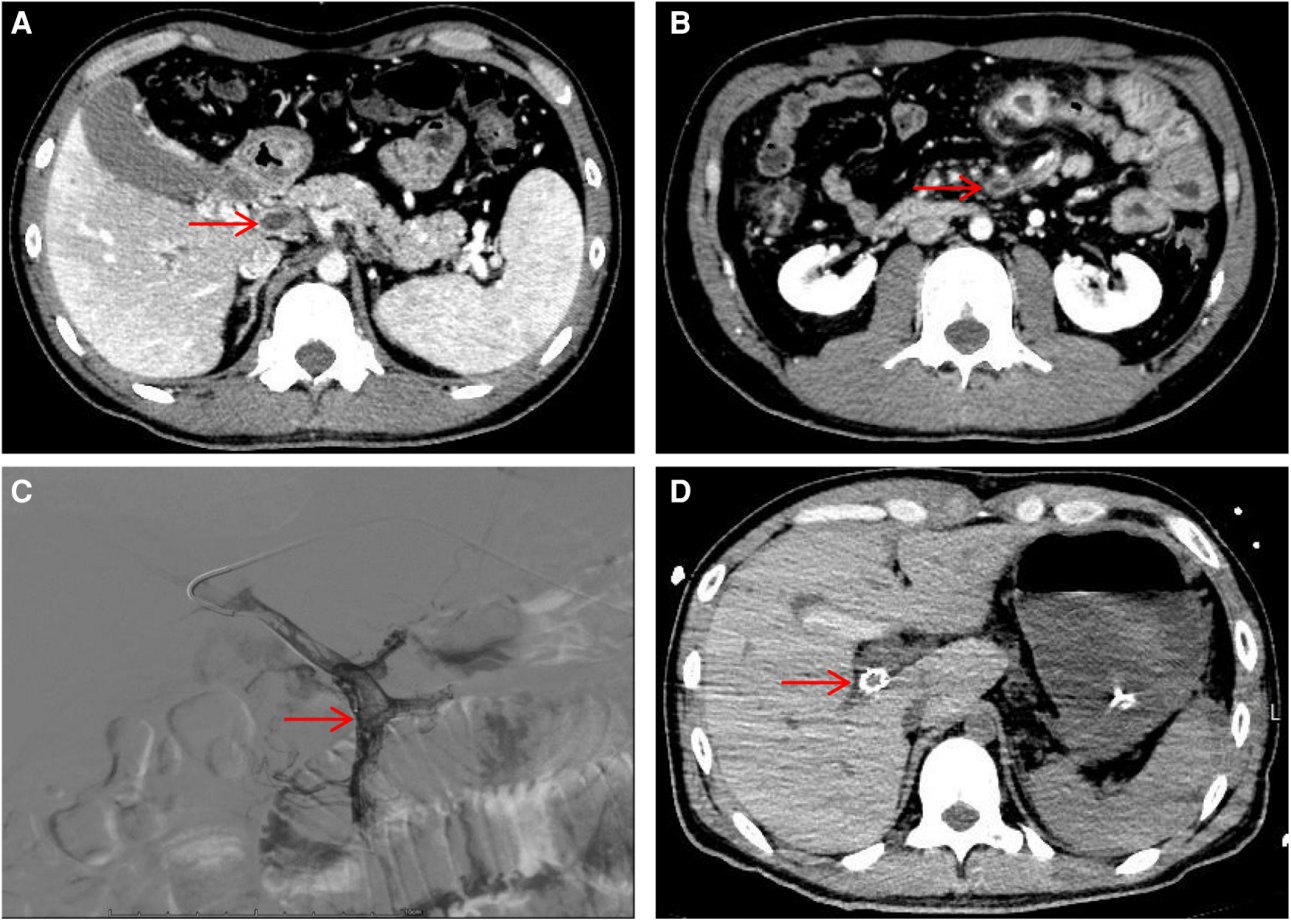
Hepatic portal vein CT angiography and digital subtraction-angiography (DSA). (A) Showing portal vein thrombosis (red arrow). (B) Showing superior mesenteric vein thrombosis (red arrow). (C) DSA showed filling defects in the portal and superior mesenteric veins. (D) Visualization of stent shadow after TIPS.
